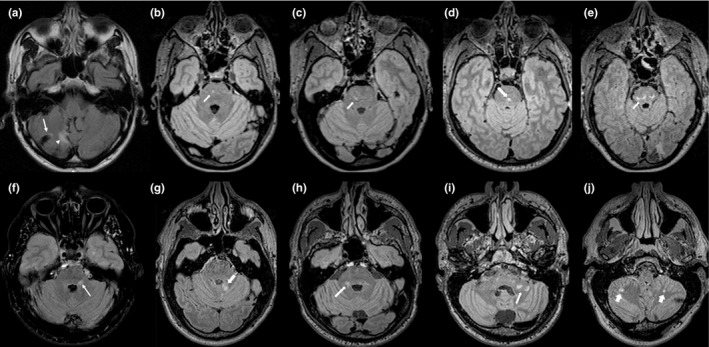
Axial FLAIR images showing the different patterns of infratentorial lesions in five patients with Fabry disease (a–e) and five subjects affected by Multiple Sclerosis (f–j). In FD, a typical vascular appearance is present (a), with the preferential involvement of the central portions of the pons (b‐e). On the other hand, in MS infratentorial lesions resemble classic demyelinating plaques, with the characteristic involvement of the middle cerebellar peduncles (f–i) or the cerebellar white matter (j)
